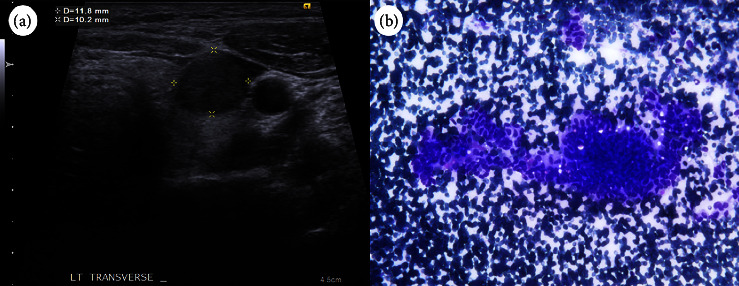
(a) Thyroid ultrasonography images depicting a solid, hypoechoic nodule with a well-defined border on the left lobe. (b) Fine-needle aspiration cytology findings revealing numerous spindle-shaped tall cells with a densely packed structure and an enlarged nucleus-to-cytoplasm ratio.
